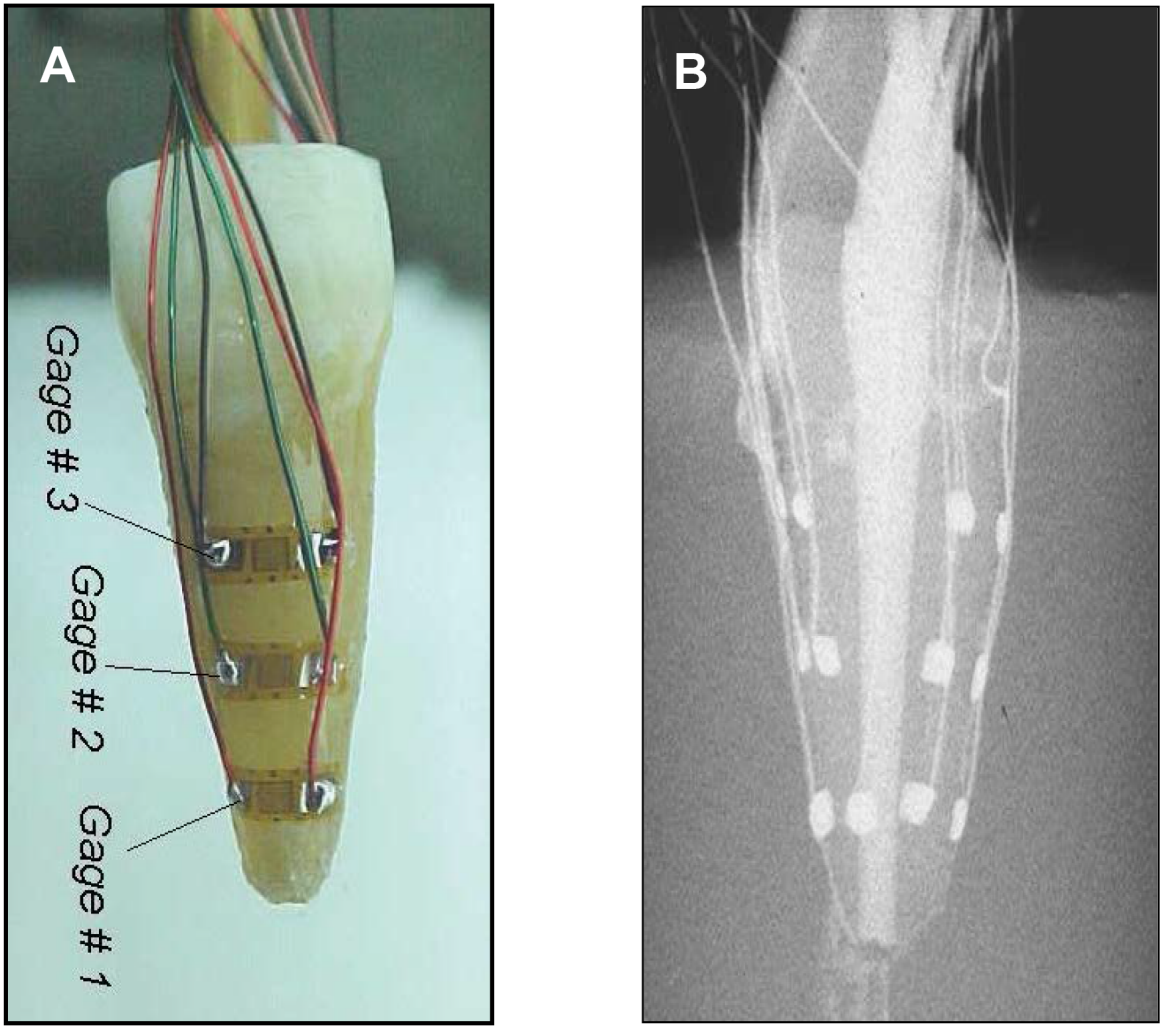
The experimental setup. (A) Maxillary central incisor with 6 miniature strain gauges mounted and wired according to configuration A. Three buccal gauges are shown (the three lingual gauges are not shown in the image). (B) Radiograph of the maxillary central incisor at the end of the lateral compaction procedure demonstrating the 6 mounted and wired strain gauges (mesial-distal view).
